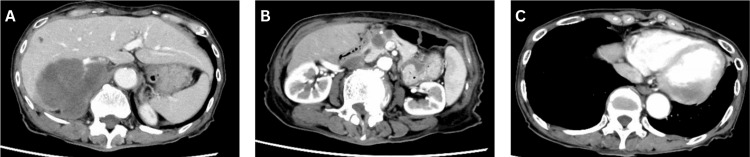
Whole-body CT images of the liver, pancreas, and cardiac sections Contrast-enhanced CT showed multiple hypovascular lesions in the liver (A), pancreas (B) and heart (C). CT: computed tomography
